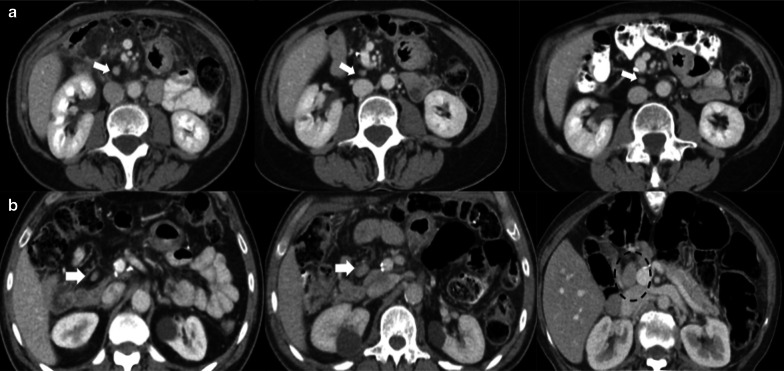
Lymphadenopathy vs. Tumor recurrence. CT challenge. Two different patients (a) 63-year-old female patient with persisting lymph node along 2-year follow-up. Six months, one- and two-years CT scans, respectively. Note the decreasing size of the node over time (big arrow). Clinical and biological markers level stable. (b) 65-year-old male patient along annual CT scan controls. An increasing lymph node is seen during the follow-up (large arrow). Note the rounded shape appearance and the short axis size greater than 10 mm (dotted circle) after three years from surgery. Tumor recurrence was proven by biopsy in patient B
